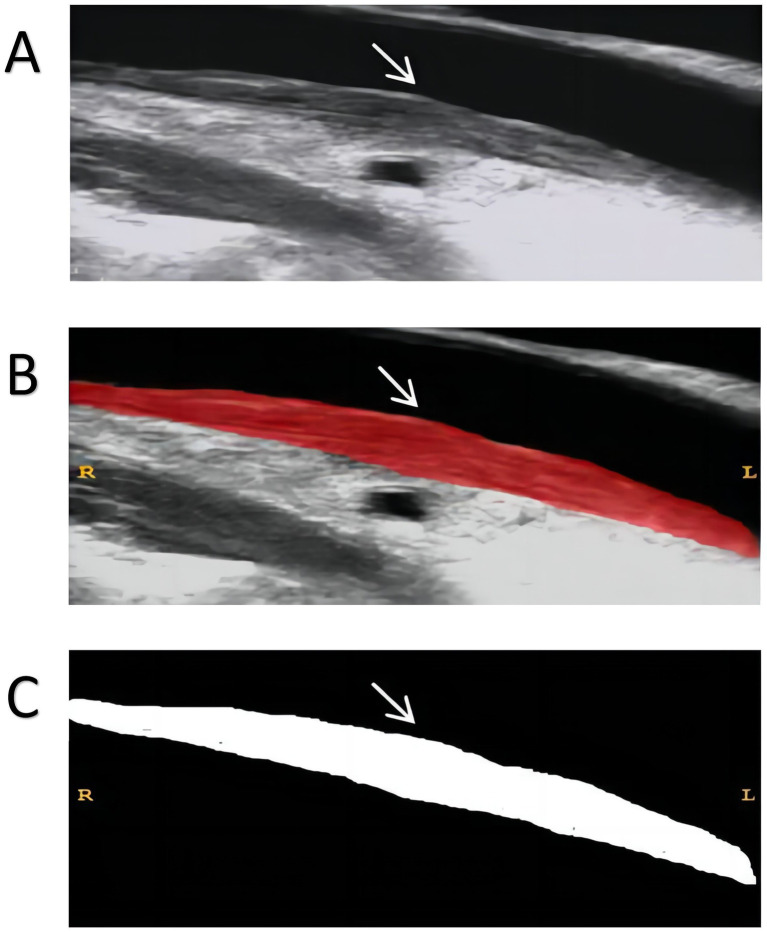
Example of manually segmented CDU images. (A) Original ultrasound image of the plaque. (B) The segmentation region was adjusted along the maximum longitudinal cross-section of the plaque. (C) ITK-SNAP software was used to label the segmented plaque image for further analysis.
